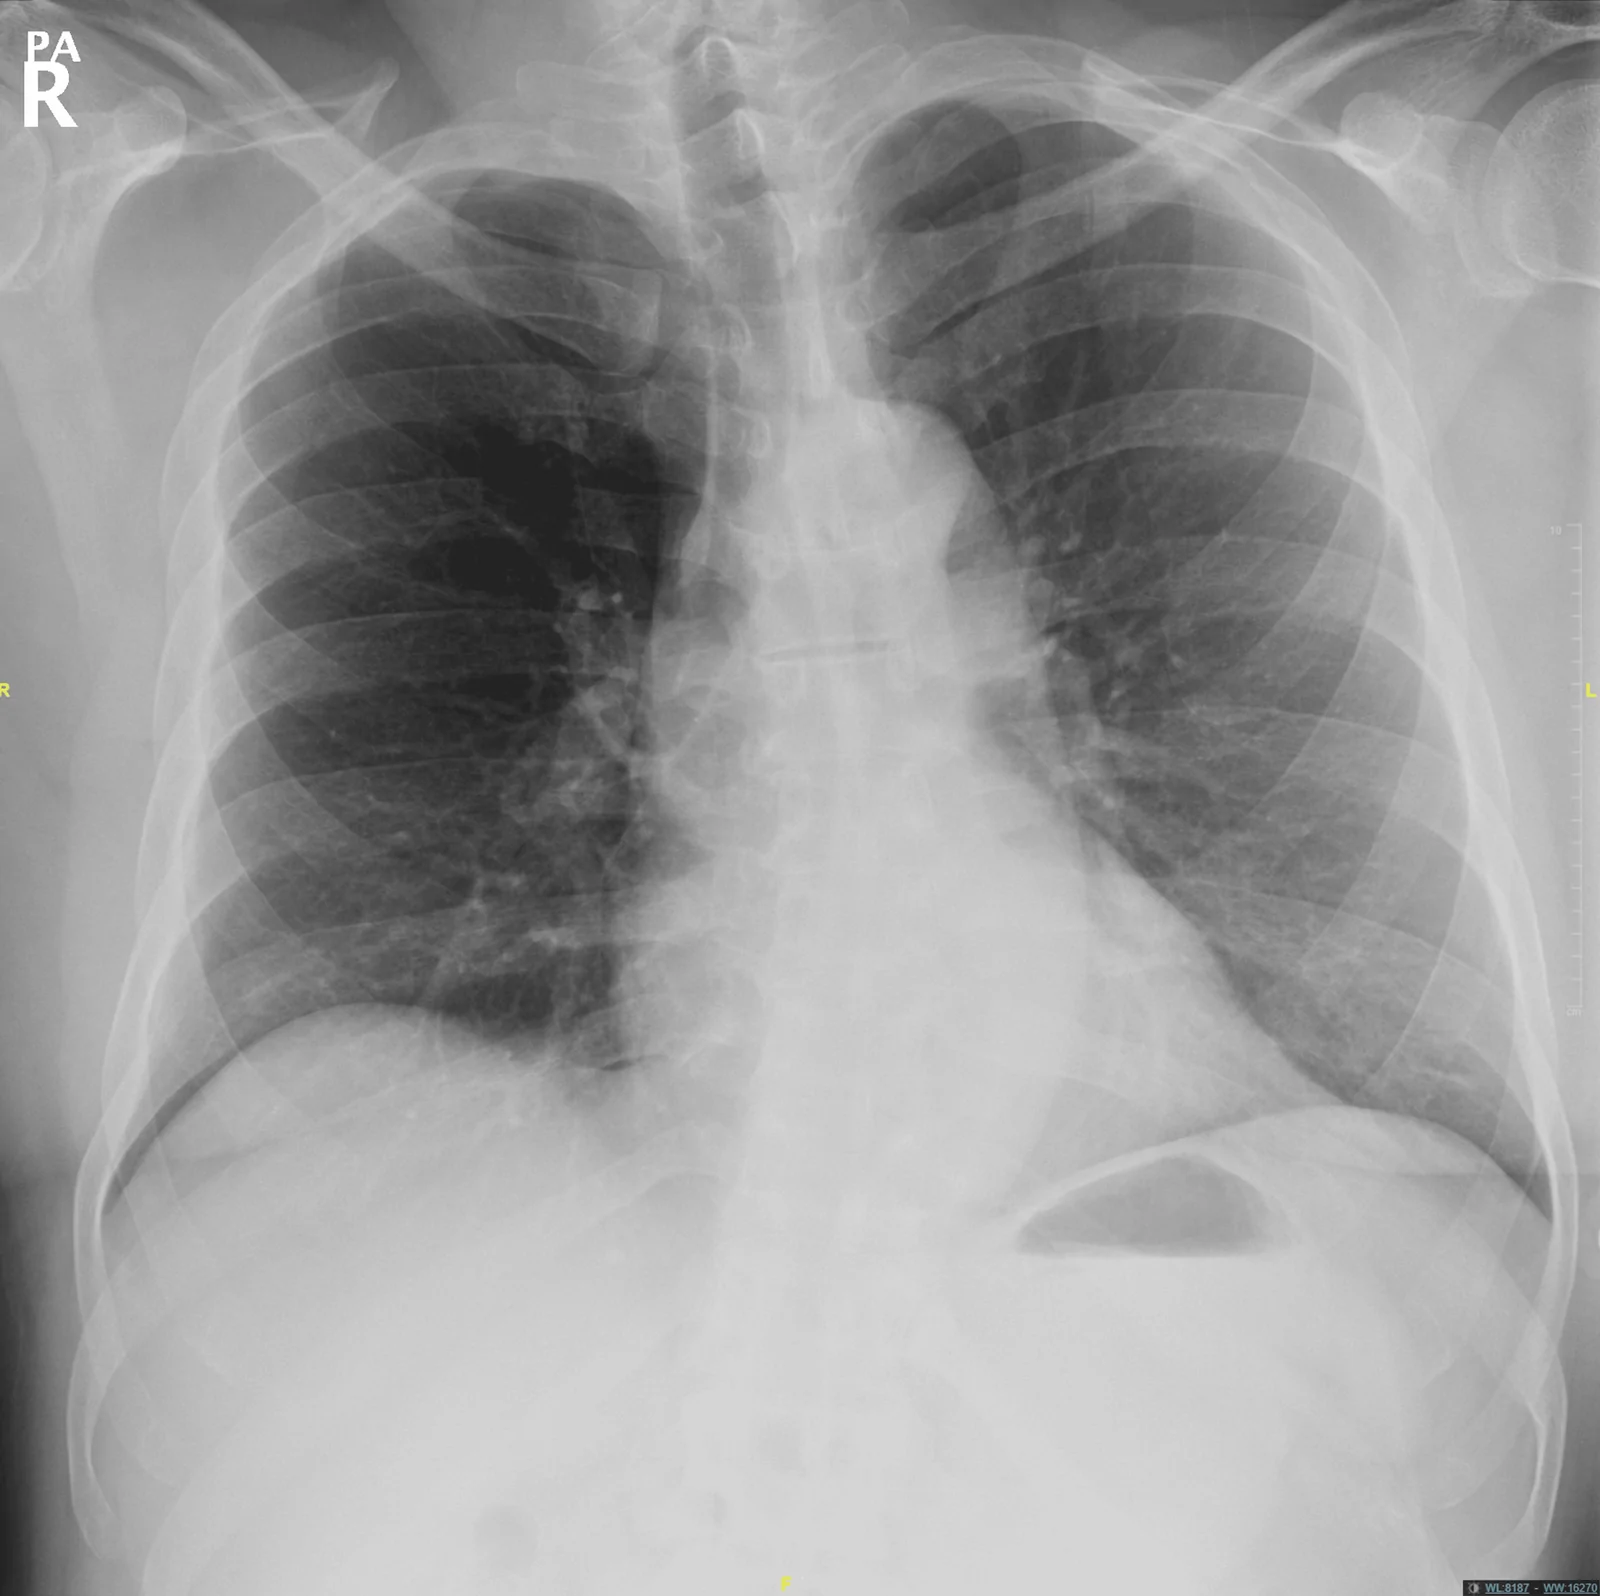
Chest radiograph obtained during the initial evaluation demonstrating no focal pulmonary infiltrates, pleural effusion, or other acute cardiopulmonary abnormalities.
